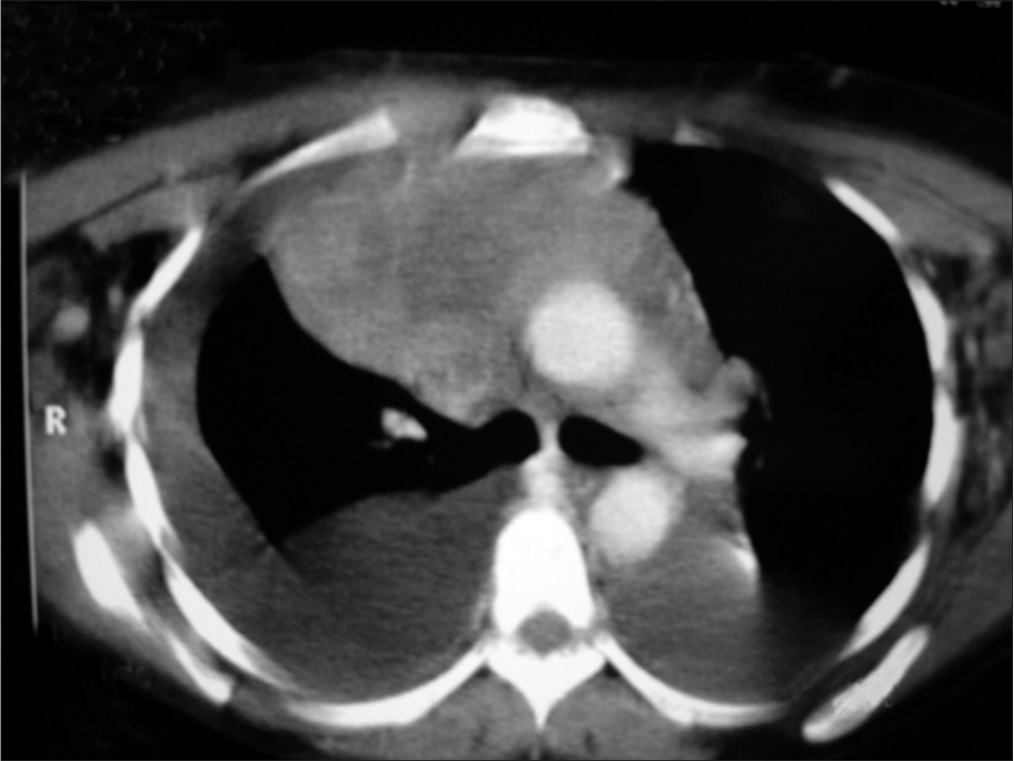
CT of thorax showing bilateral pleural effusion with large anterior mediastinal mass and superior vena cava thrombosis
